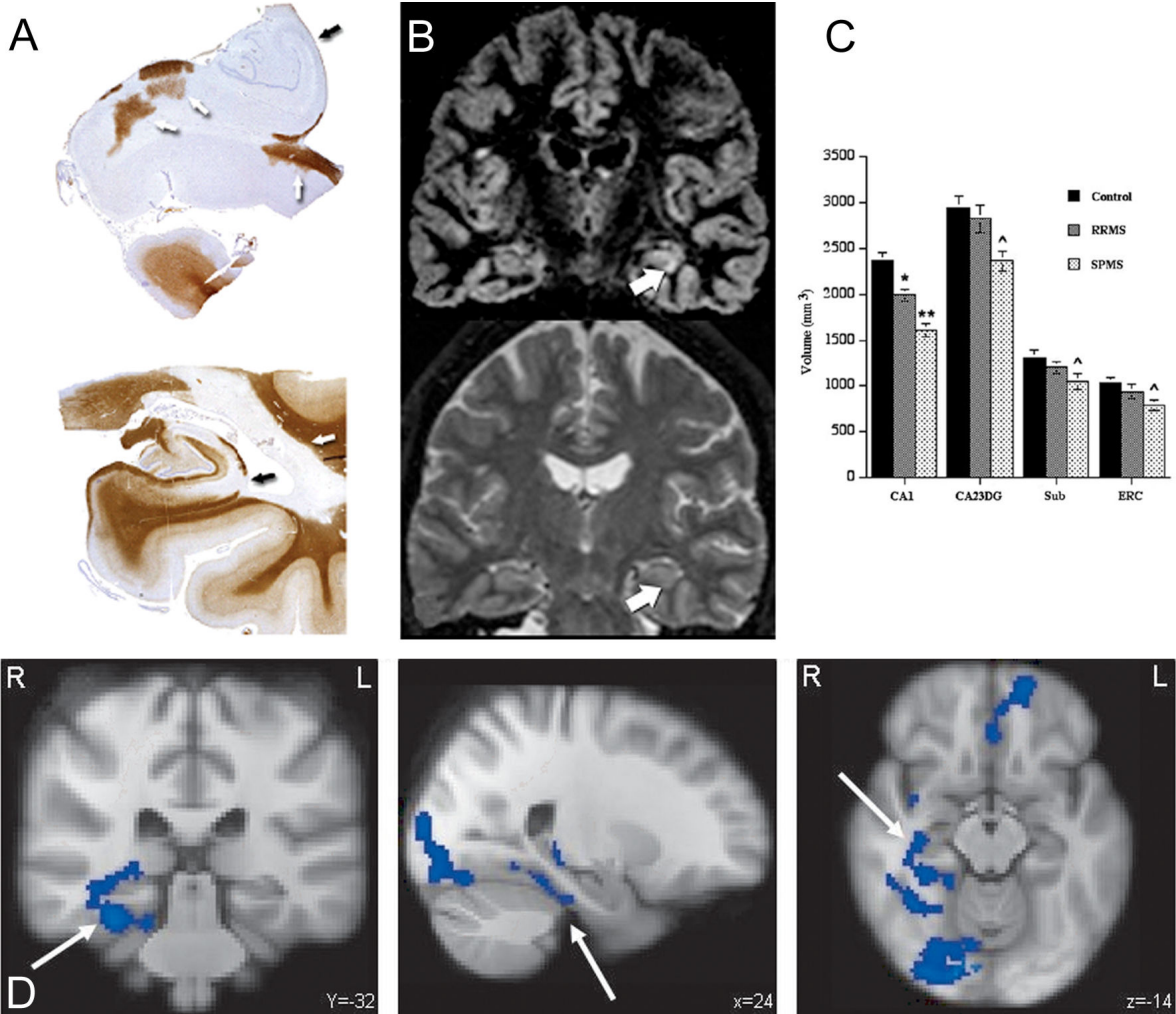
Non-neocortical GM damage: the hippocampus. A) Extensive hippocampal demyelination observed in post-mortem material. Reproduced from Geurts et al. [95] with permission from the American Association of Neuropathologists Inc. B) In vivo detection of hippocampal lesions using DIR imaging. Reproduced from Roosendaal et al. [116] with permission from John Wiley and Sons. C) Hippocampal atrophy on high field MRI provides detailed information on the specific location in the hippocampus where atrophy is present. Reproduced from Sicotte et al. [118] with permission from Oxford University Press. D) Anno 2011 it is besides structural changes also possible to study functional changes in the hippocampus; the blue areas indicates reduced hippocampal activity in cognitively impaired MS patients compared to healthy controls. Reproduced from Hulst et al. [127] with permission from John Wiley and Sons.
